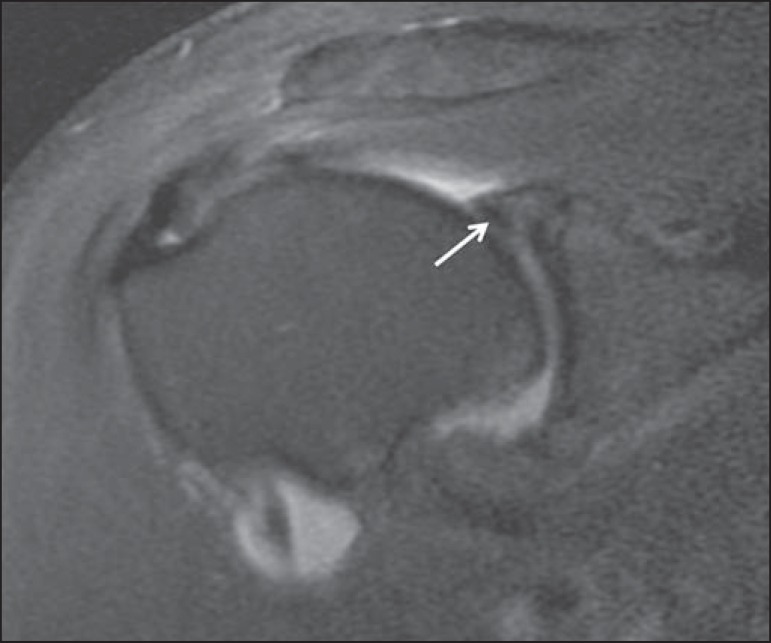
56-year-old male. Coronal T2-weighted MRI scan with fat saturation. The
